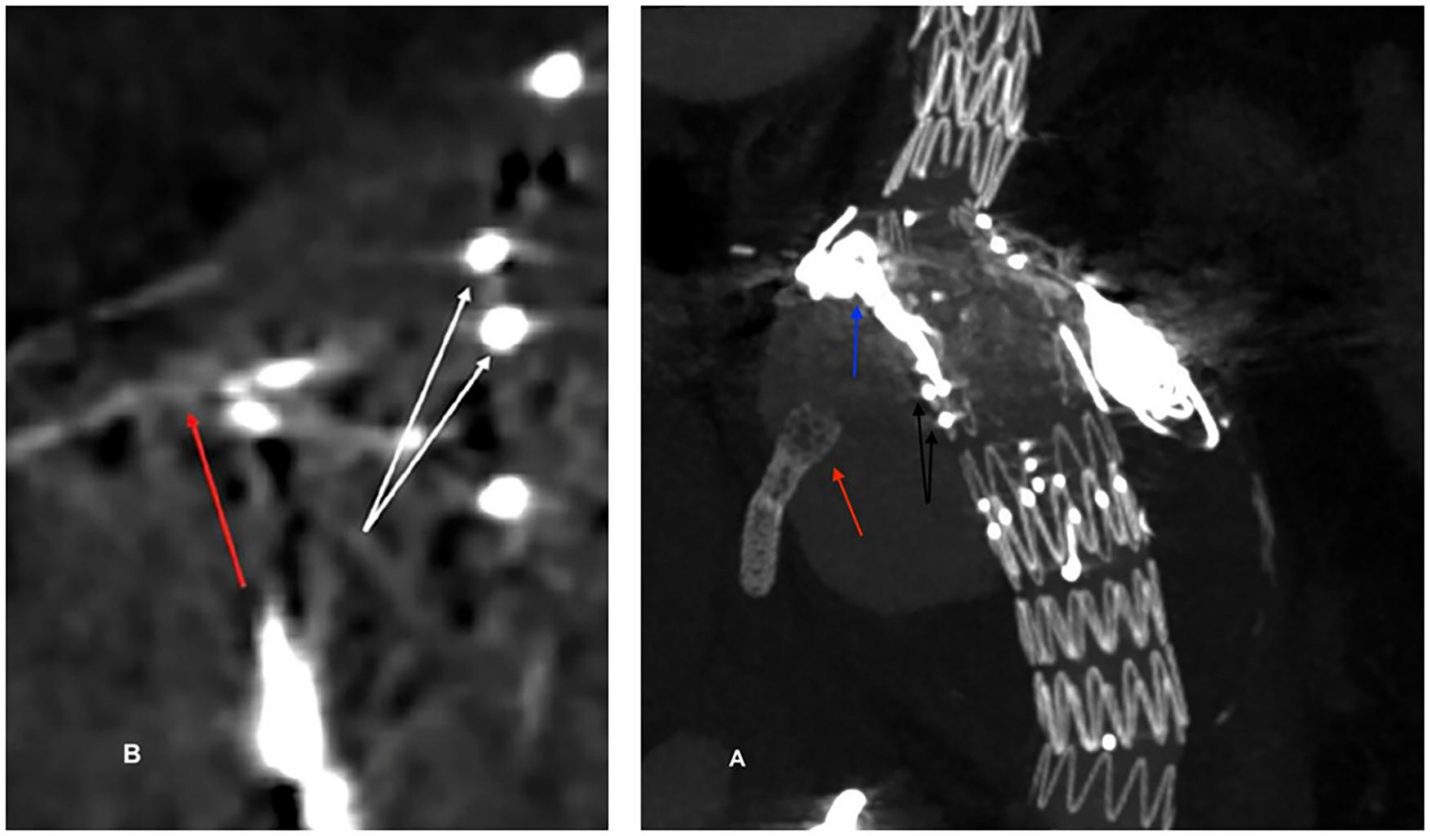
3D reconstruction of the preoperative CT scan. (A) Type IIIB endoleak due to a disconnection between the stent (red arrow) and the fenestration (black arrows) of the RRA. The coils within the aneurysmal sac are also visible (blue arrow). (B) Type IIIB/IIIC endoleak due to a disconnection and fracture between the stent (red arrow) and the fenestration (white arrows) of the SMA. RRA, right renal artery; SMA, superior mesenteric artery.
